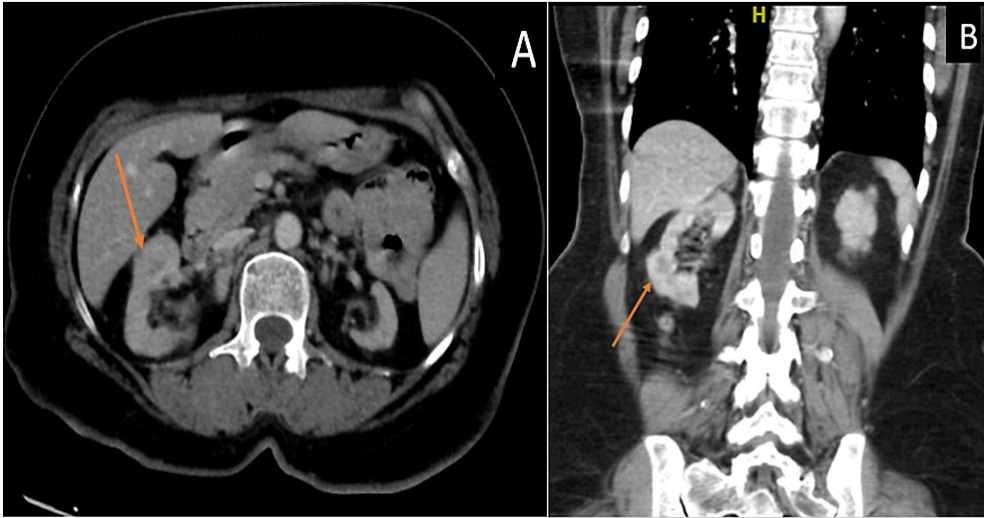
A & B: Contrast-enhanced axial and coronal CT scan images of the abdomen showing a heterogenous slightly hypodense mass of the right kidney (arrow).
